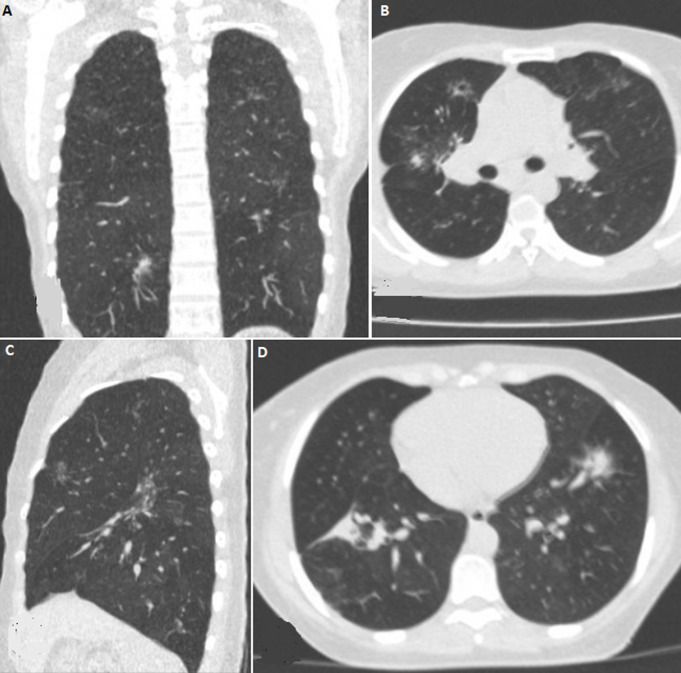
non-contrast Chest CT with deep inspiration in lung window: A) coronal reconstruction; B) axial section; C) sagittal reconstruction. Demonstrate a total disappearance of ground-glass opacities Persistence of retractile opacities; D) axial section
